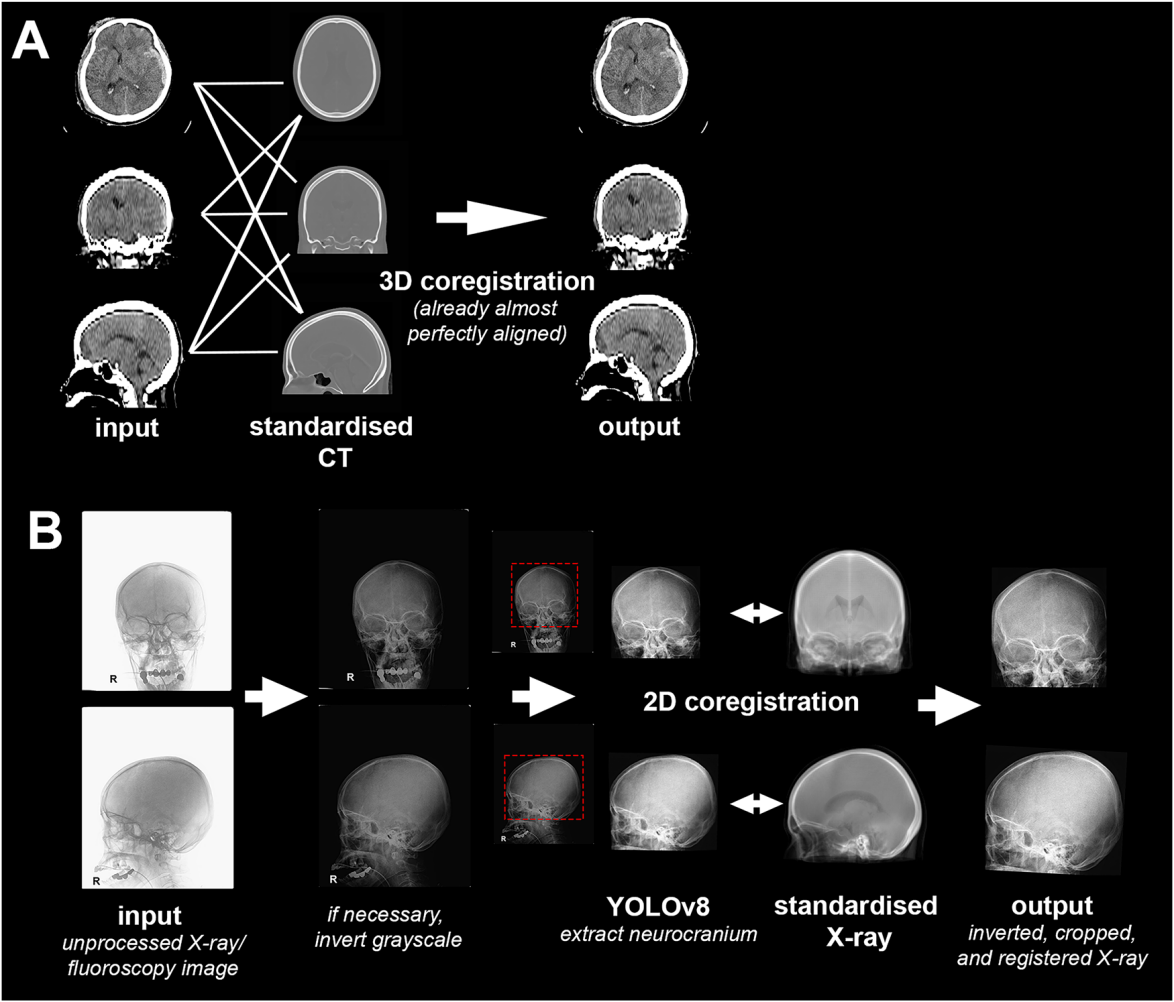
Preprocessing for model 2. A Step1: registration of a windowed CT scan to a standardized CT scan. B Steps 2, 3, and 4: Inversion of grayscale (if necessary), extraction of neurocranium, and rigid registration of patient X-rays to standardized X-rays
